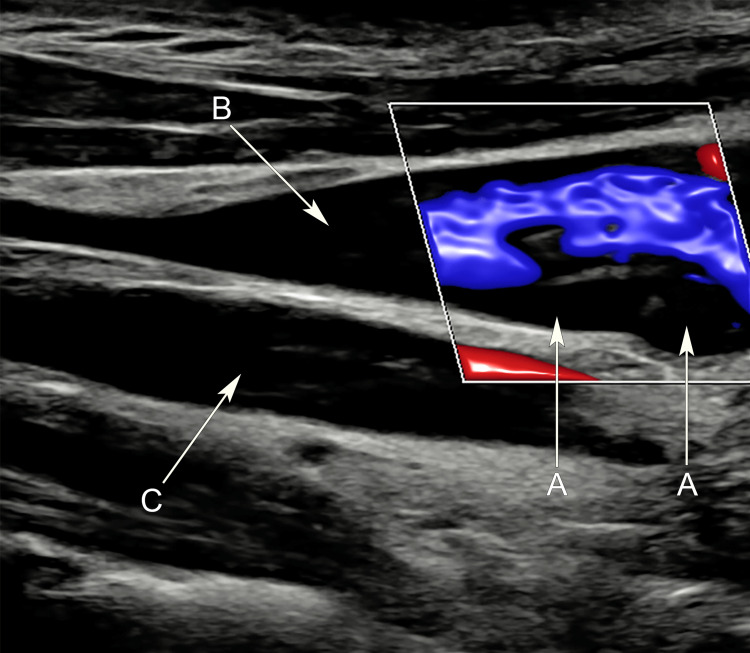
Longitudinal sonogram of the left neck Sonogram demonstrates a hypoechoic, non-occlusive thrombus within a dilated left internal jugular vein (arrow A). Patent internal jugular vein is shown cranial to the thrombus (arrow B), and common carotid artery (arrow C).
